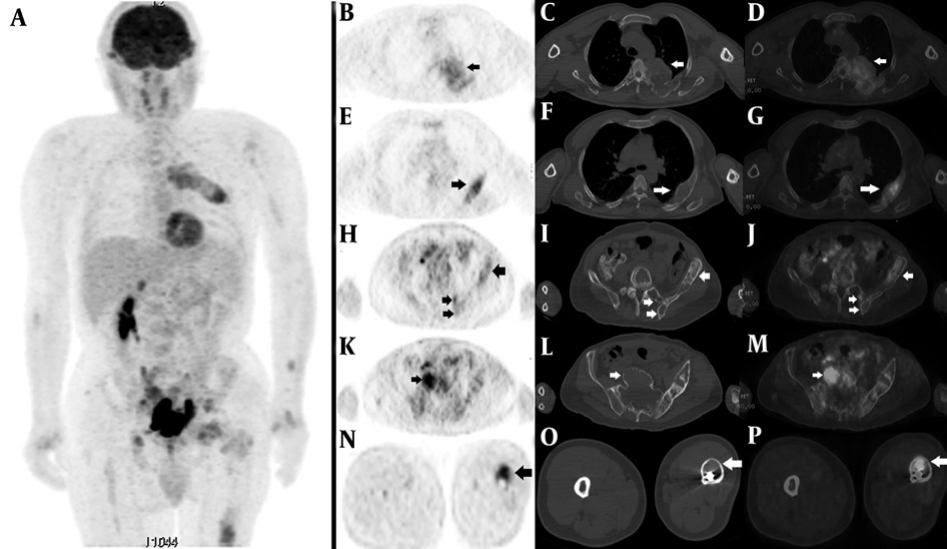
A, Maximum intensity projection (MIP) image; B, E, H, K, N: axial FDG PET images; C, F, I, L, O: axial CT images; D, G, J, M, P: axial fused FDG PET/CT images. FDG PET/CT images showed lytic, expansile, mild to moderate heterogeneous hypermetabolic bone lesions in the left hemithorax, left hemipelvis and left lower extremity.
